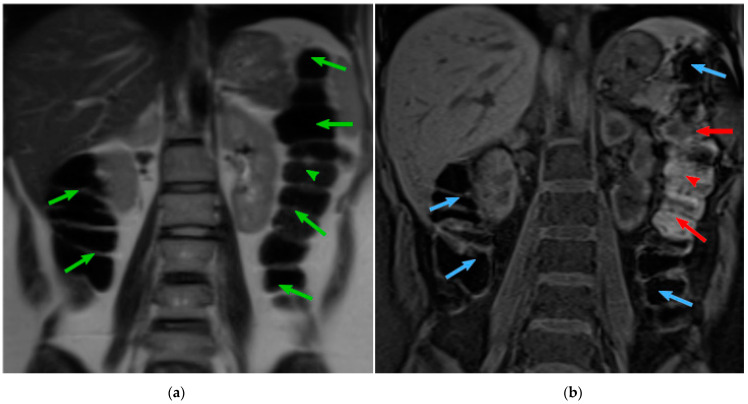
(a) MRI T2 coronal slice showing ascending colon and descending colon (green arrows). (b) The same area in the T1-FS modality. Arrows are colorized depending on the contents: blue arrows point to gas and red to feces. Courtesy of [7].
